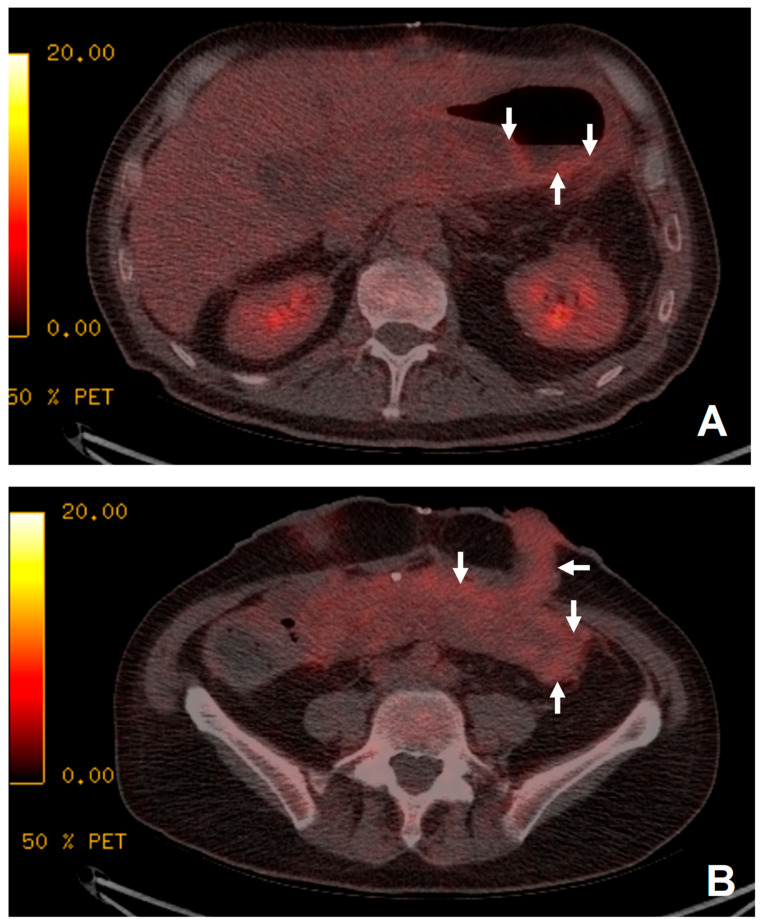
The patient presented with 1 week of pyrexia but no source of infection was identified. Increased uptake was demonstrated in the transplanted stomach (white arrows, image (A)) and small bowel (white arrows, image (B)) suggestive of rejection. Biopsy just prior to ^18^F-FDG PET/CT demonstrated a non-specific increase in apoptotic debris within the lamina propria of uncertain significance. As no focus of infection was demonstrated and the appearance of the transplanted stomach (A) and small bowel (B) was suggestive of rejection, the patient was treated for rejection with immune suppression. Following anti-rejection treatment, the patient’s clinical picture improved with resolution of the pyrexia. Subsequent biopsies taken 6 weeks later demonstrated no remaining features of rejection.
